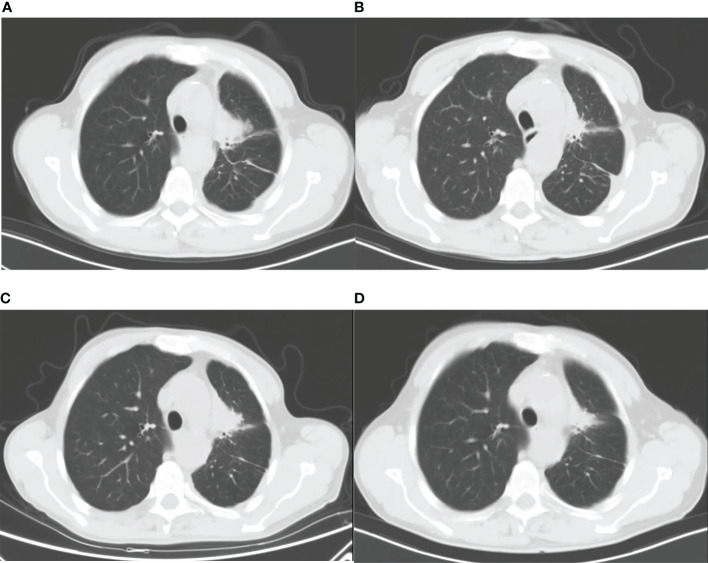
Lung CT images: (A) before dacomitinib administration; (B) 37 days after dacomitinib administration; (C) 50 days after dacomitinib termination; and (D) 95 days after afatinib administration.
